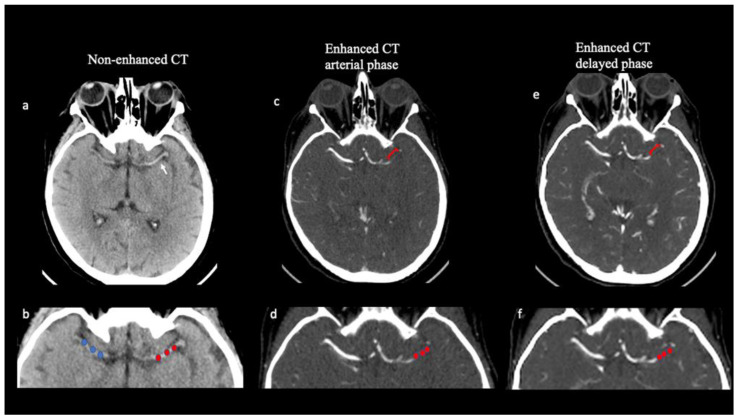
Thrombus attenuation measurements and thrombus perviousness quantification. Axial non-contrast CT (NCCT; a,b) demonstrates a hyperattenuating (“dense”) middle cerebral artery (MCA) (white arrow in a) in a 67-year-old patient with acute stroke. Thrombus attenuation measurement was calculated on NCCT as the difference between thrombus average density and contralateral artery by placing 3 spherical ROIs in the proximal, middle, and distal parts of the thrombus in the M1 segment of the left middle cerebral artery (red ROIs in b) and along the same vessel on the contralateral side (blue ROIs in b). Multiphase CTA (mCTA; c–f) identifies occlusion length of the M1 segment of the left middle cerebral artery, demonstrated as contrast-filling defects (square bracket in c,e). Three regions of interest (red ROIs) were placed in a left middle cerebral artery thrombus both in the arterial (c,d) and in the delayed phases (e,f) of the mCTA. Clot perviousness measurement was calculated as the difference in average thrombus attenuation measured on the arterial or delayed phase of mCTA and on NCCT.
